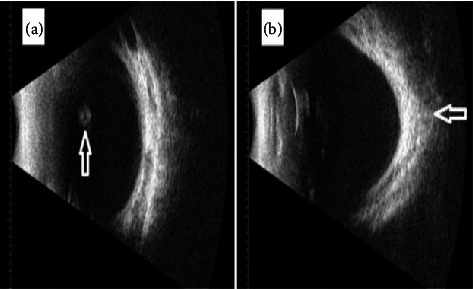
B-scan ultrasound of the left eye. (a) A floating cyst in the anterior vitreous cavity and (b) the posterior staphyloma (as highlighted in the two arrows).
